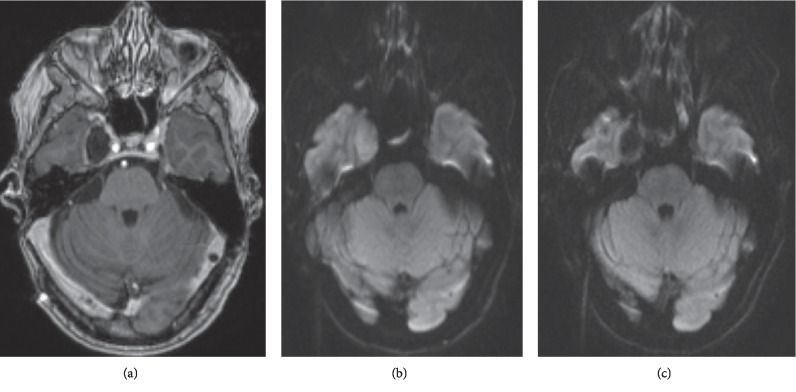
Imaging of patient 1. (a) Preoperative contrast-enhanced axial T1 MRI showing peripheral enhancement of the cyst wall. (b) Diffusion restriction noted in the lesion preoperatively. (c) Postoperative resolution of diffusion restriction within the lesion.
